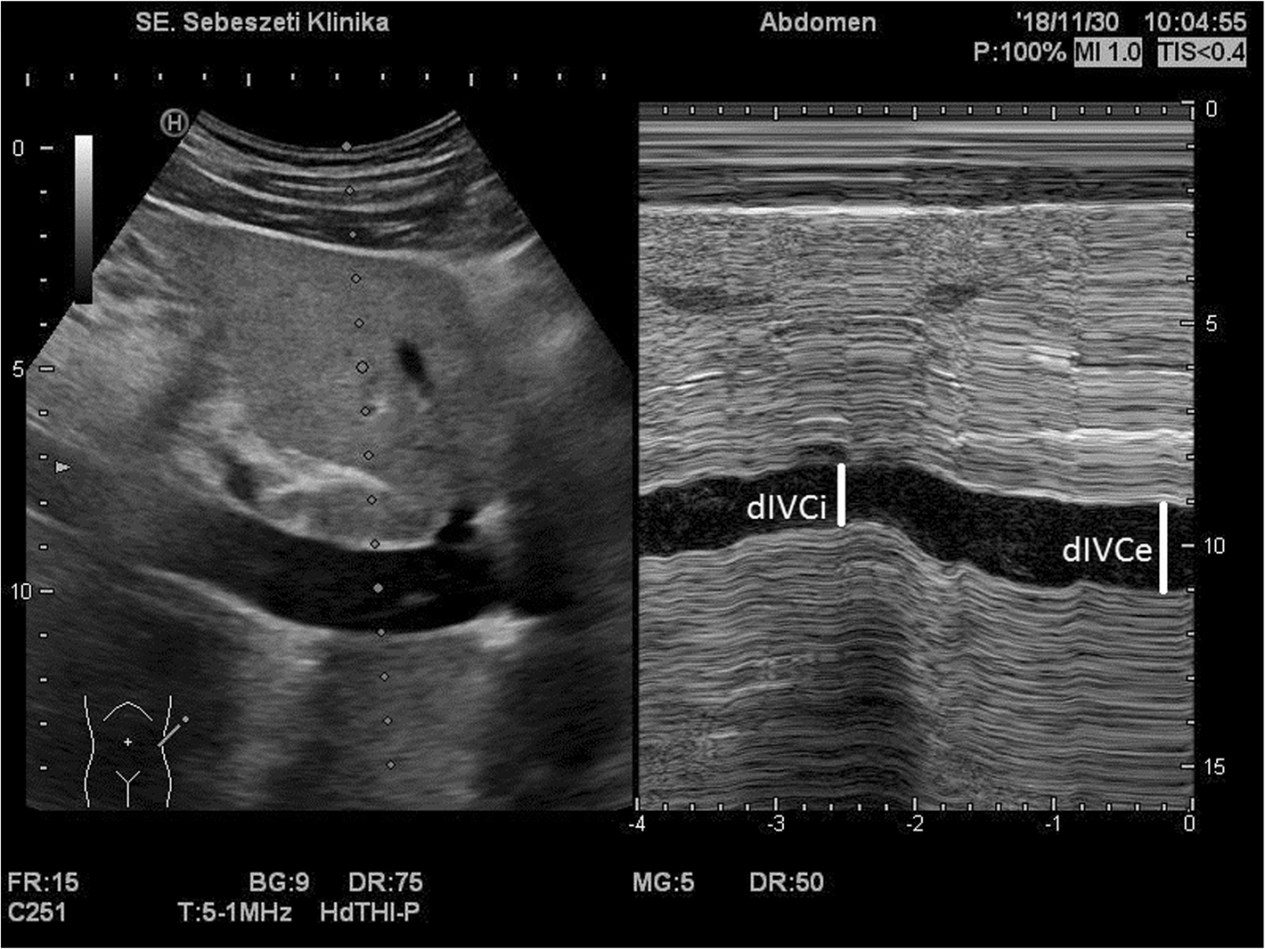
Typical ultrasound image of the inferior vena cava near the heart. M-mode image represents high respiratory collapsibility. (dIVCe = IVC diameter in expiration, dIVCi = IVC diameter in inspiration)
